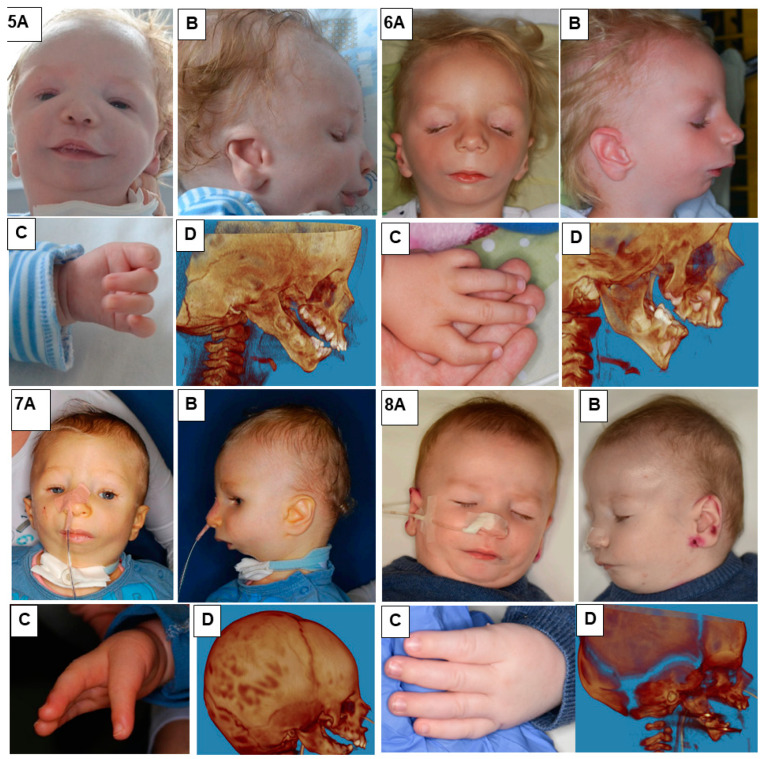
Patients with Nager syndrome: (5) newborn, (6) three years of age, (7) two years of age, and (8) two years of age. (A): front view. (B): lateral view. (C): (5) view of hand with residual undeveloped thumb, (6) hand with an incorrectly positioned and underdeveloped thumb, and (7,8) hand with congenital absence of a thumb. (D): 3D reconstruction of a CT image lateral projection from before treatment.
